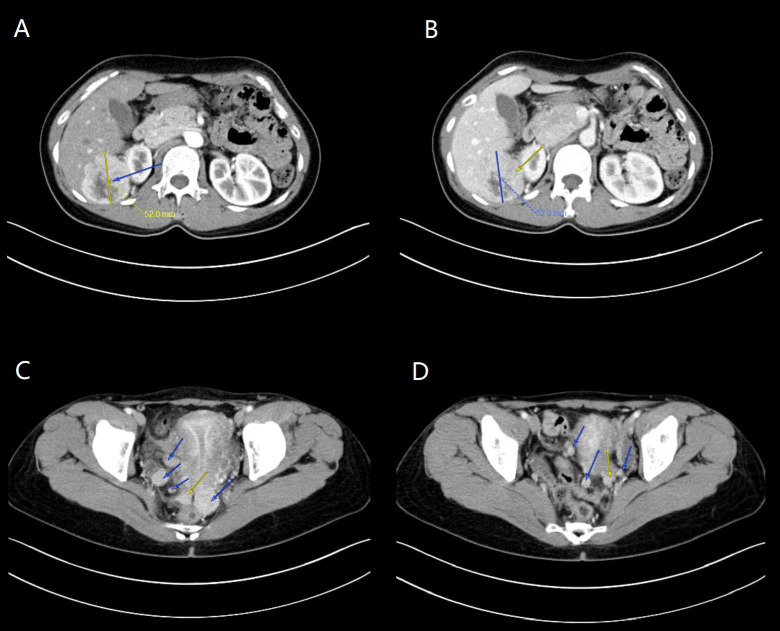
The enhanced CT examination revealed the multiple metastatic lesions in abdominopelvic cavity. (A,B, arrow) the largest tumor in right hepatorenal recess; (C,D, arrow) multiple seedings of tumors in pelvic cavity.
